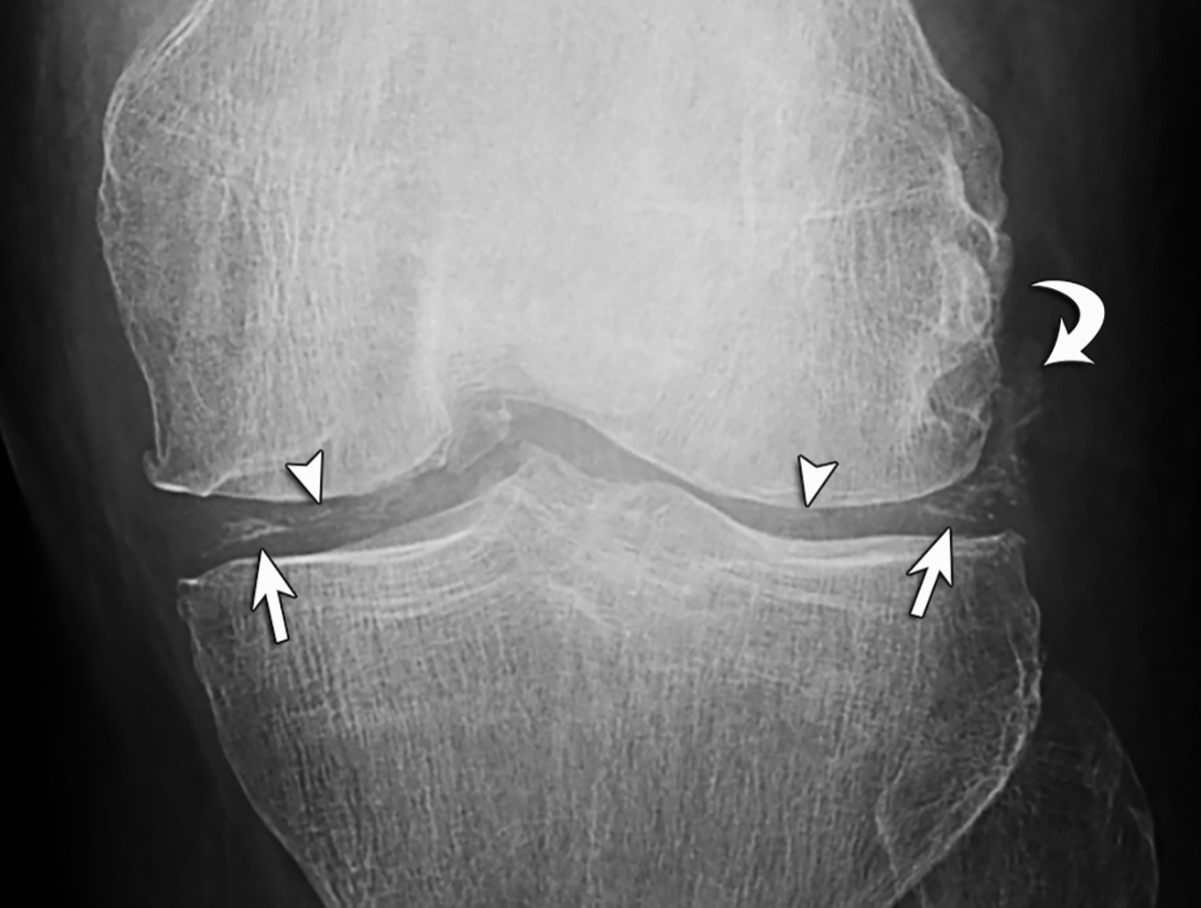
82-year-old woman with CPPD. Anteroposterior knee radiograph shows cartilage icing with linear opacity outlining of the menisci and hyaline cartilage (arrows). Chondrocalcinosis appears as speckled calcifications within the hyaline cartilage (arrowheads). Note amorphous opacity characterized as a tophus (curved arrow) at the popliteus groove.
